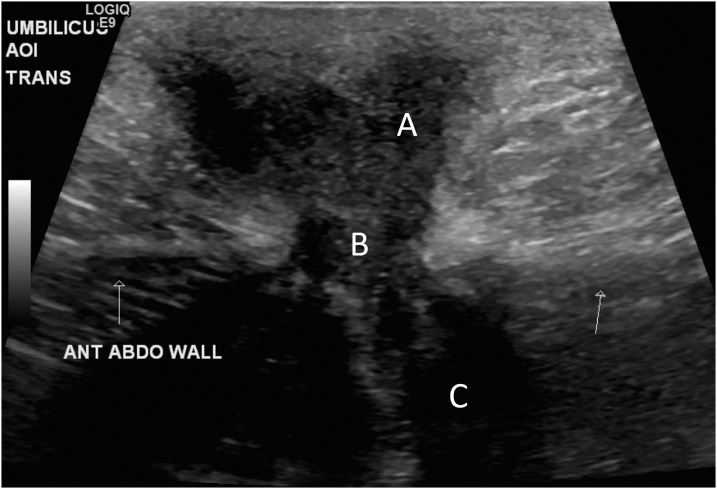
Ultrasound demonstrating hypoechoic fluid collection in the anterior abdominal wall (labelled A), communicating via a defect (labelled B), with an underlying hyperechoic structure (labelled C).
